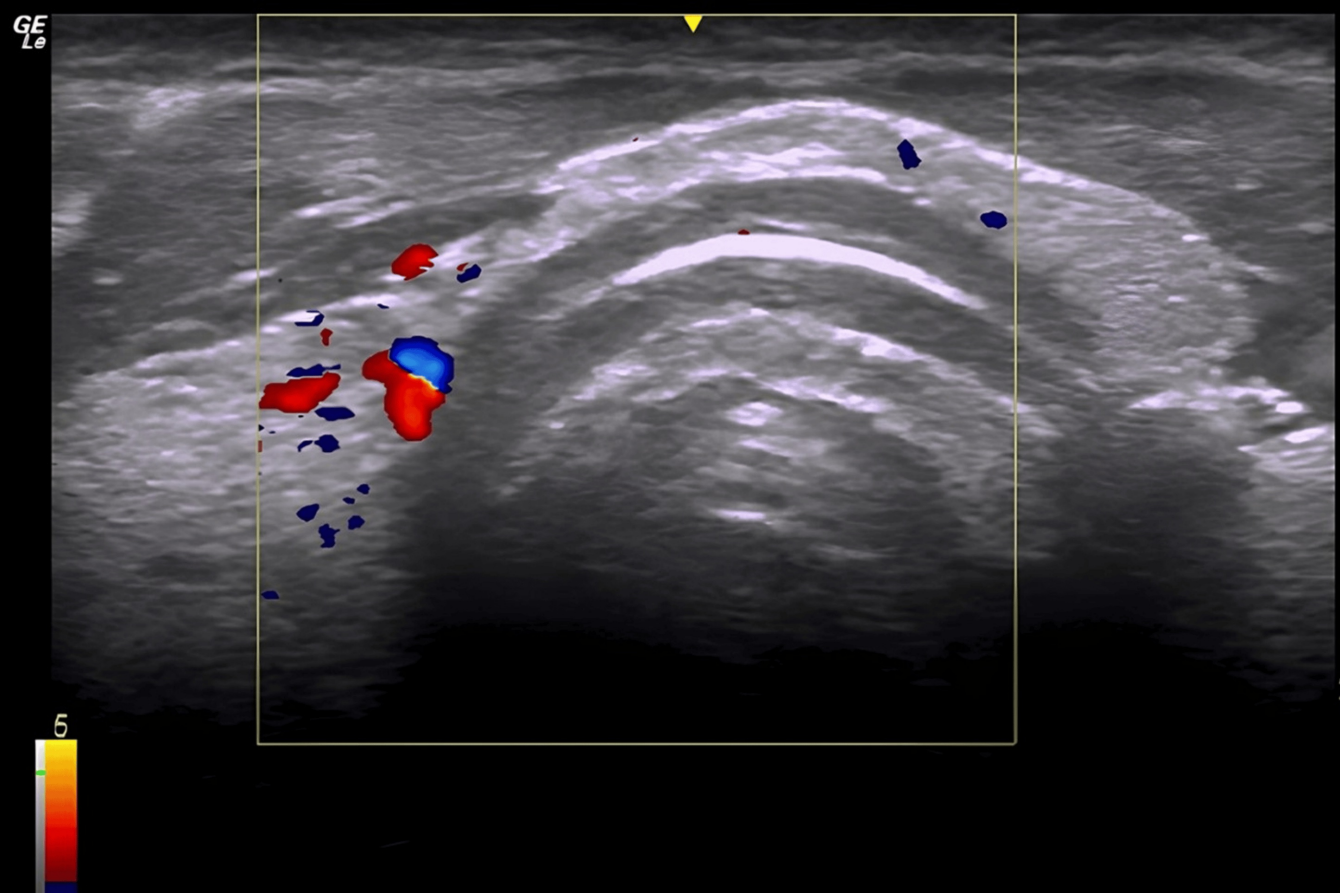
Transverse ultrasound image showing vessels around thyroid gland.
